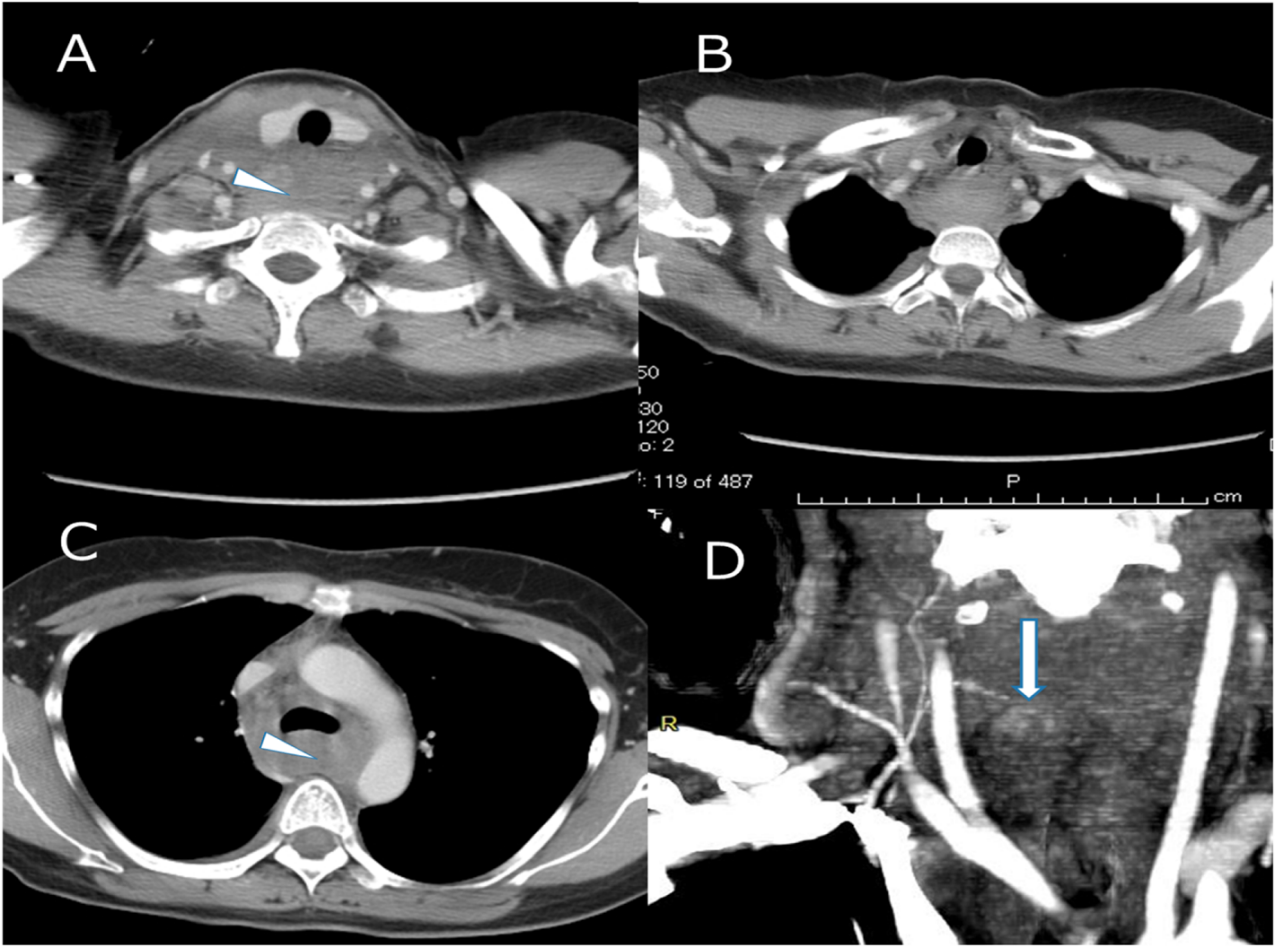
CT findings. a A cervical hematoma in the retropharyngeal space. b Mediastinal hematoma. c A mediastinal hematoma compressing the trachea, esophagus, and superior vena cava. d Extravasation of contrast medium (arrow)
